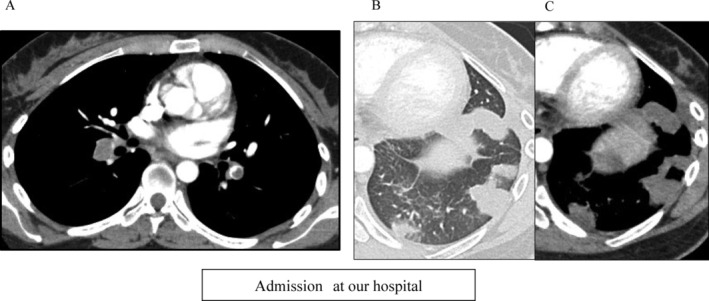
Contrast‐enhanced computed tomography images obtained at our hospital. (A) Mediastinal window setting. Pulmonary emboli are seen in the bilateral inferior pulmonary artery. (B) Lung window setting. Some well‐demarcated air space consolidation lesions are seen in the left lower lobe. One of the consolidation lesions shows central lucency. (C) Mediastinal window setting. The central lucency is better seen on mediastinal window setting
